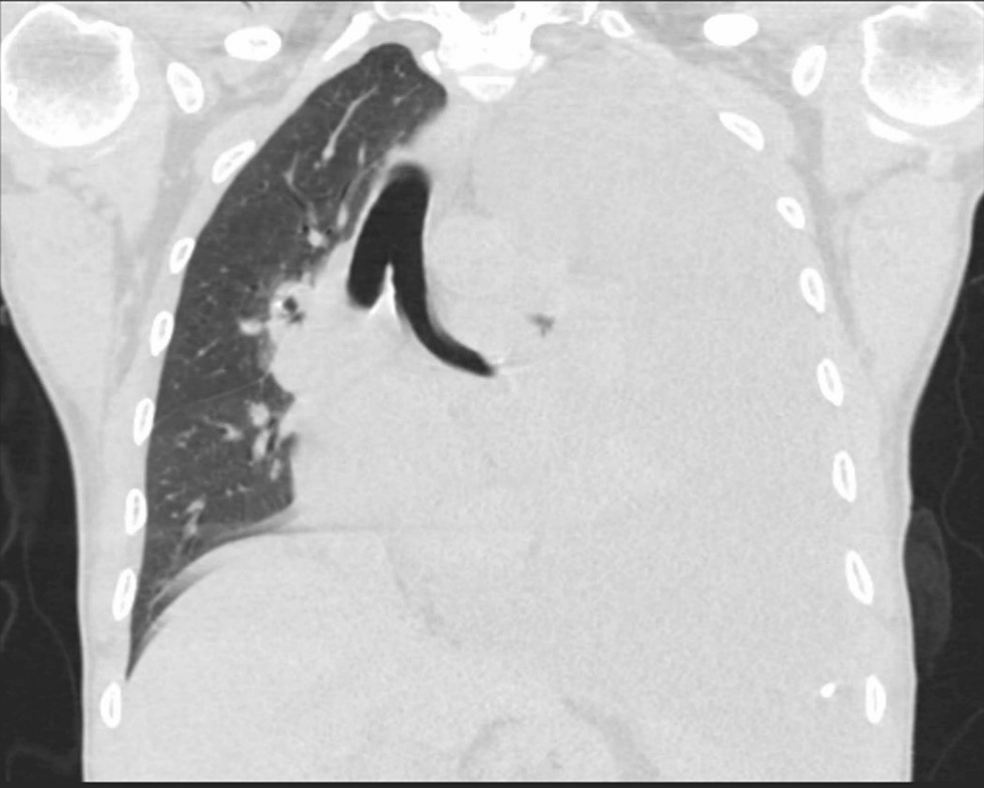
CT chest demonstrating large left-sided pleural effusion with mass effect pushing the mediastinal structures to the right side (coronal view)
